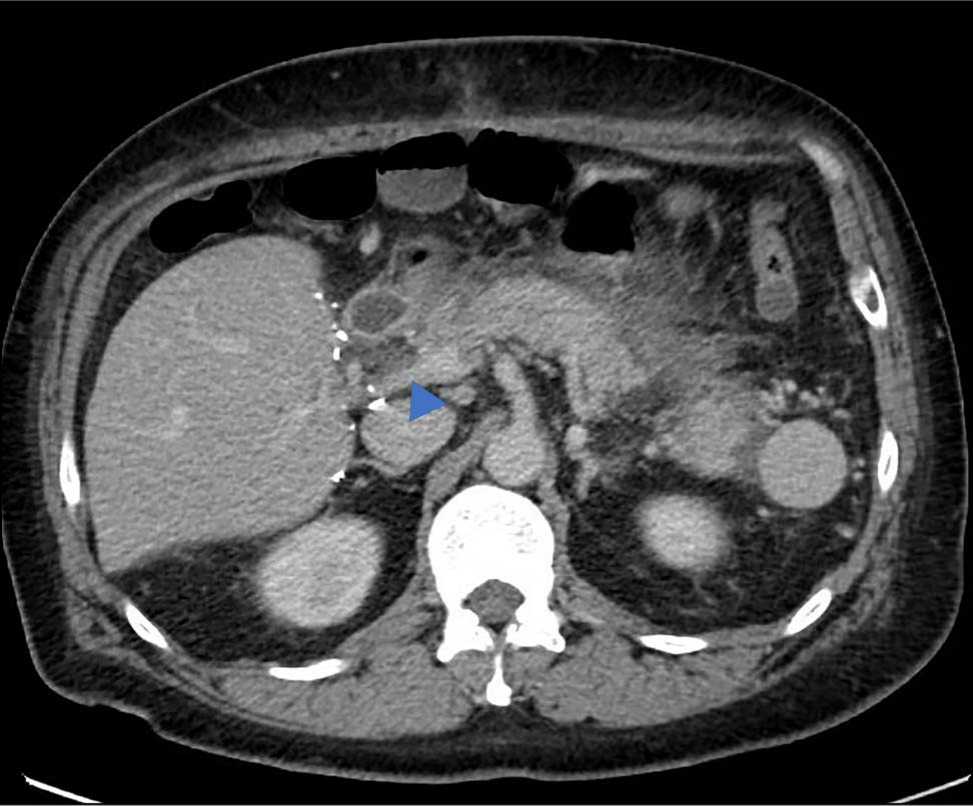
Abdominal computed tomography showing inflammation and fat stranding surrounding the pancreas and a small fluid collection of 2.0 × 1.2 cm in the pancreatic head (blue arrowhead).
